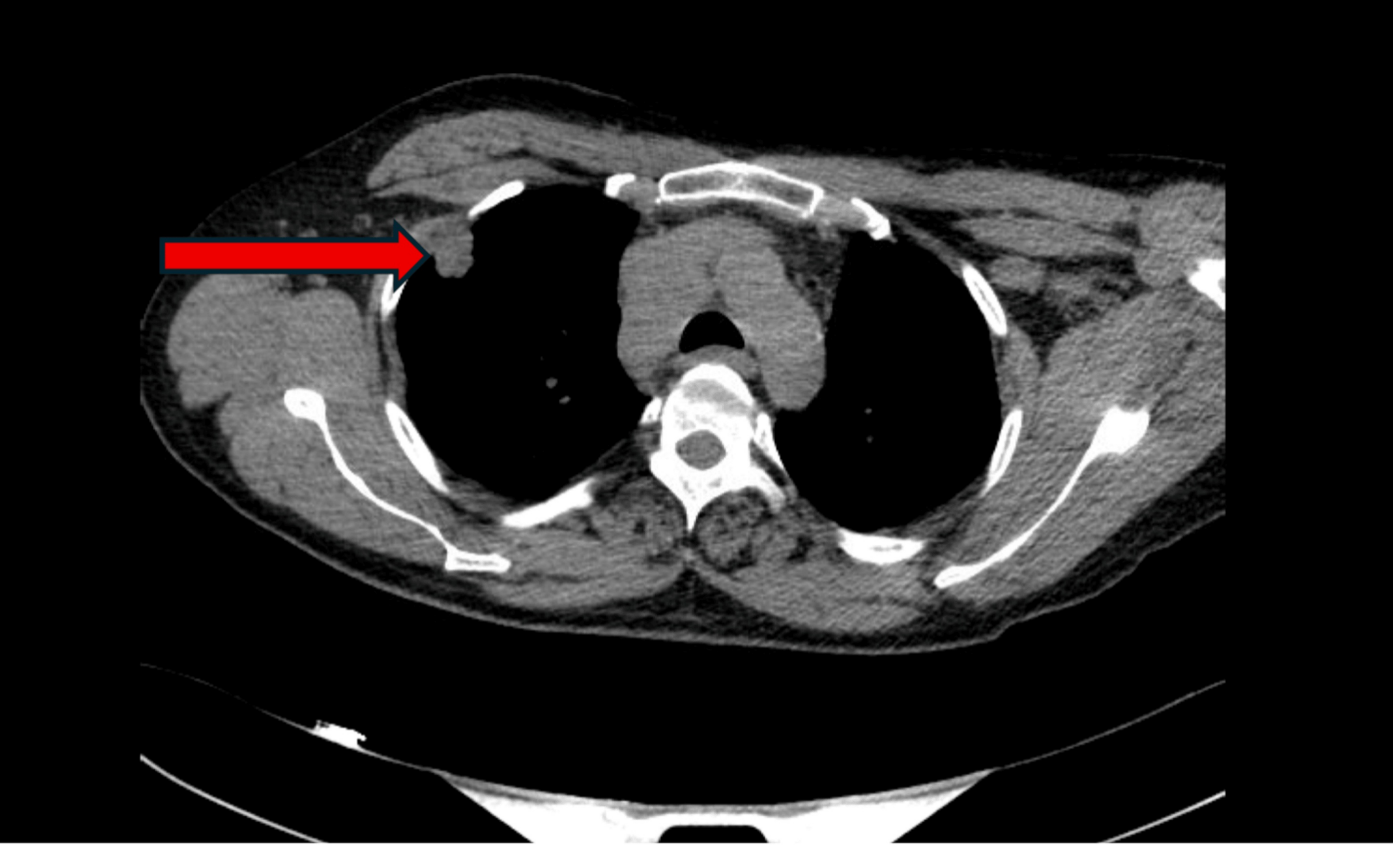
CT thorax with contrast showing a pleural-based soft-tissue opacity in the right upper lobe measuring 2.0 cm (red arrow), which was biopsied and confirmed to be small-cell lung carcinoma.
